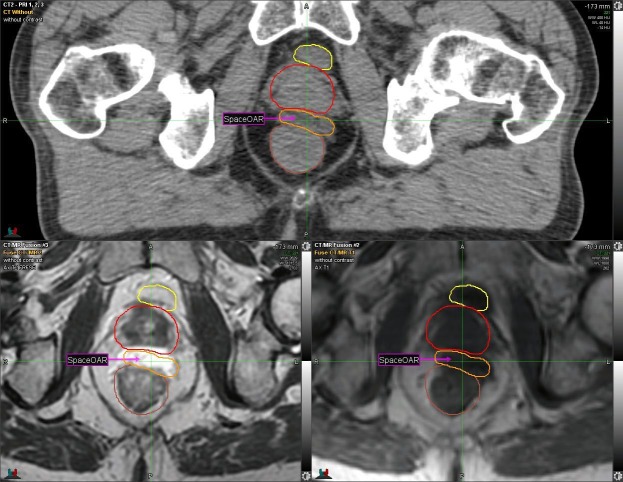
CT and MRI: Axial View Yellow = Bladder Red = Prostate Orange and Arrow = Spacer Brown = Rectum Computerized tomography: CT Magnetic resonance imaging: MRI
